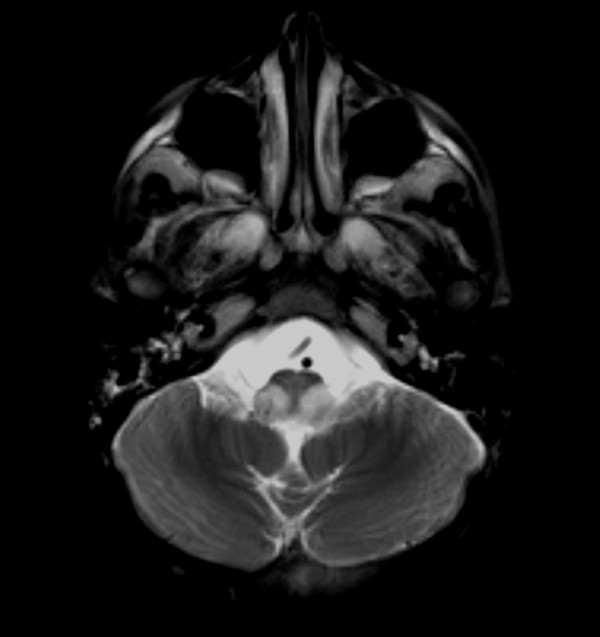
Brain computed tomography and magnetic resonance imaging at 12 months. a Computed tomography image demonstrates low attenuation areas in the white matter of the frontal lobes, putamen, external capsule and claustrum, and cystic formation in the white matter of the frontal lobes. b T2-weighted image shows areas of high signal intensity and cystic formation in the white matter of the frontal lobes, and significant deep gray matter structure abnormality. c T2-weighted image shows small, bilateral hyperintense lesions in the medulla oblongata. d Contrast T1-weighted image demonstrates contrast enhancement in the pericystic lesion and the caudate nucleus.
